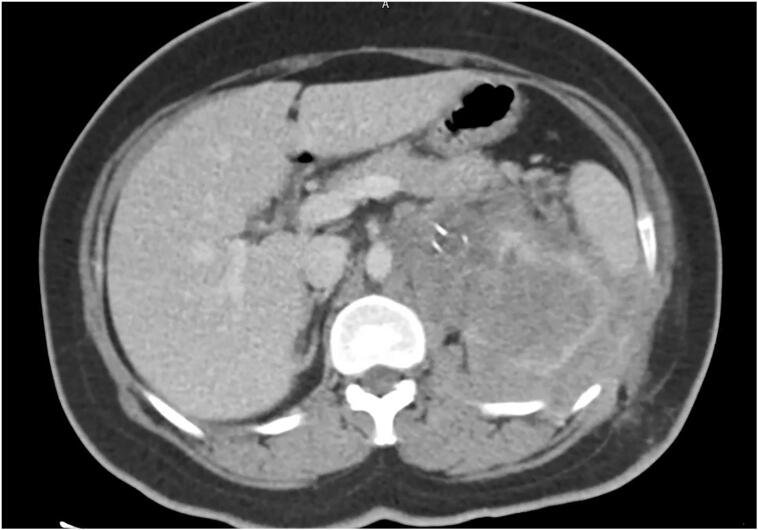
CT scan showing tumor recurrence as an infiltrative mass in the left kidney extending to renal hilum, peritoneum, psoas muscle, and abdominal wall.
